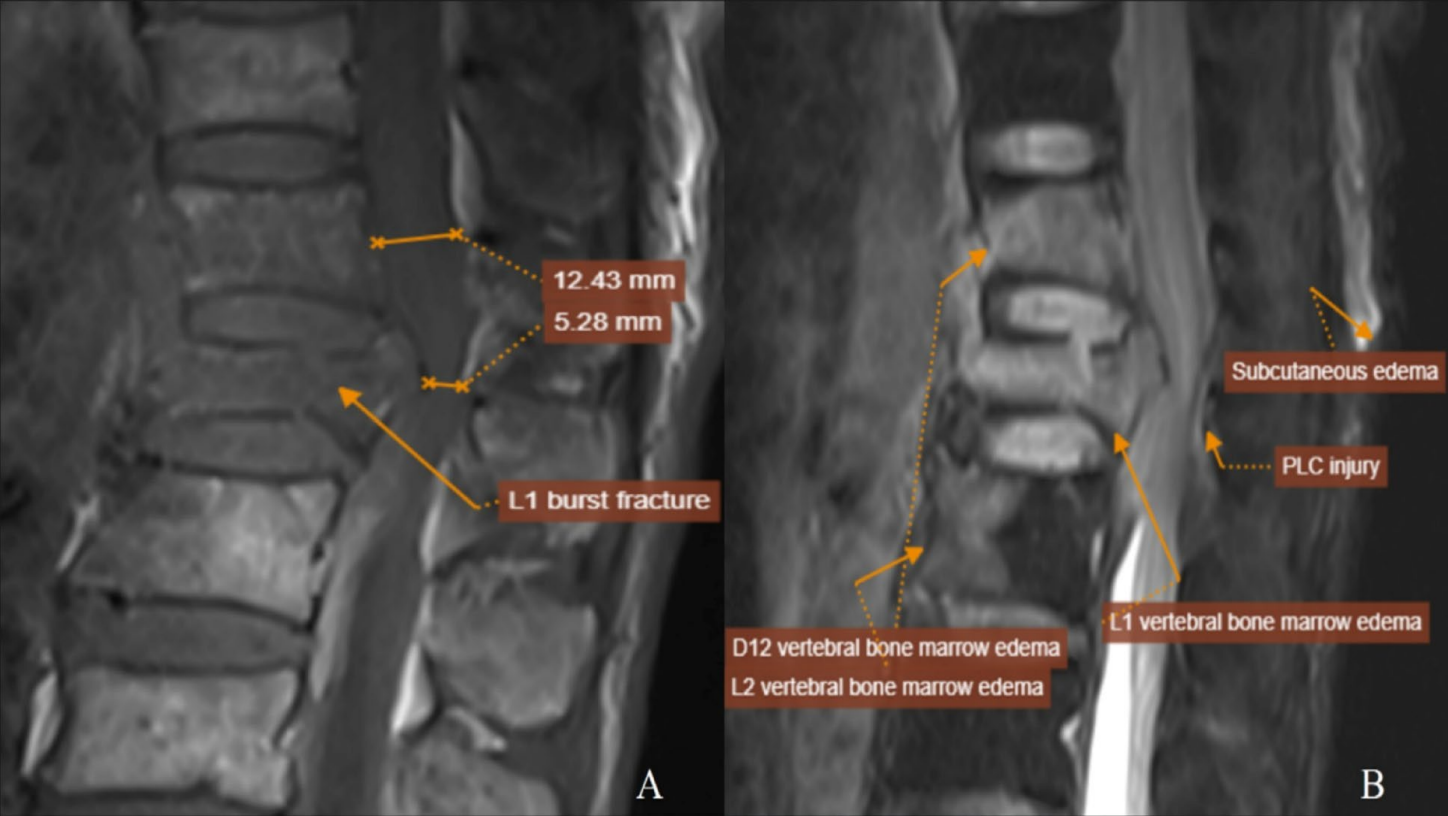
High-resolution MRI of another patient presenting with PLC disruption and severe canal compromise. MRI of L1 fracture with > 50% canal compromise (A) and PLC injury (B); mTLICS = 5 treated with posterior fixation (Images enhanced for contrast and clarity at 300 dpi resolution)
